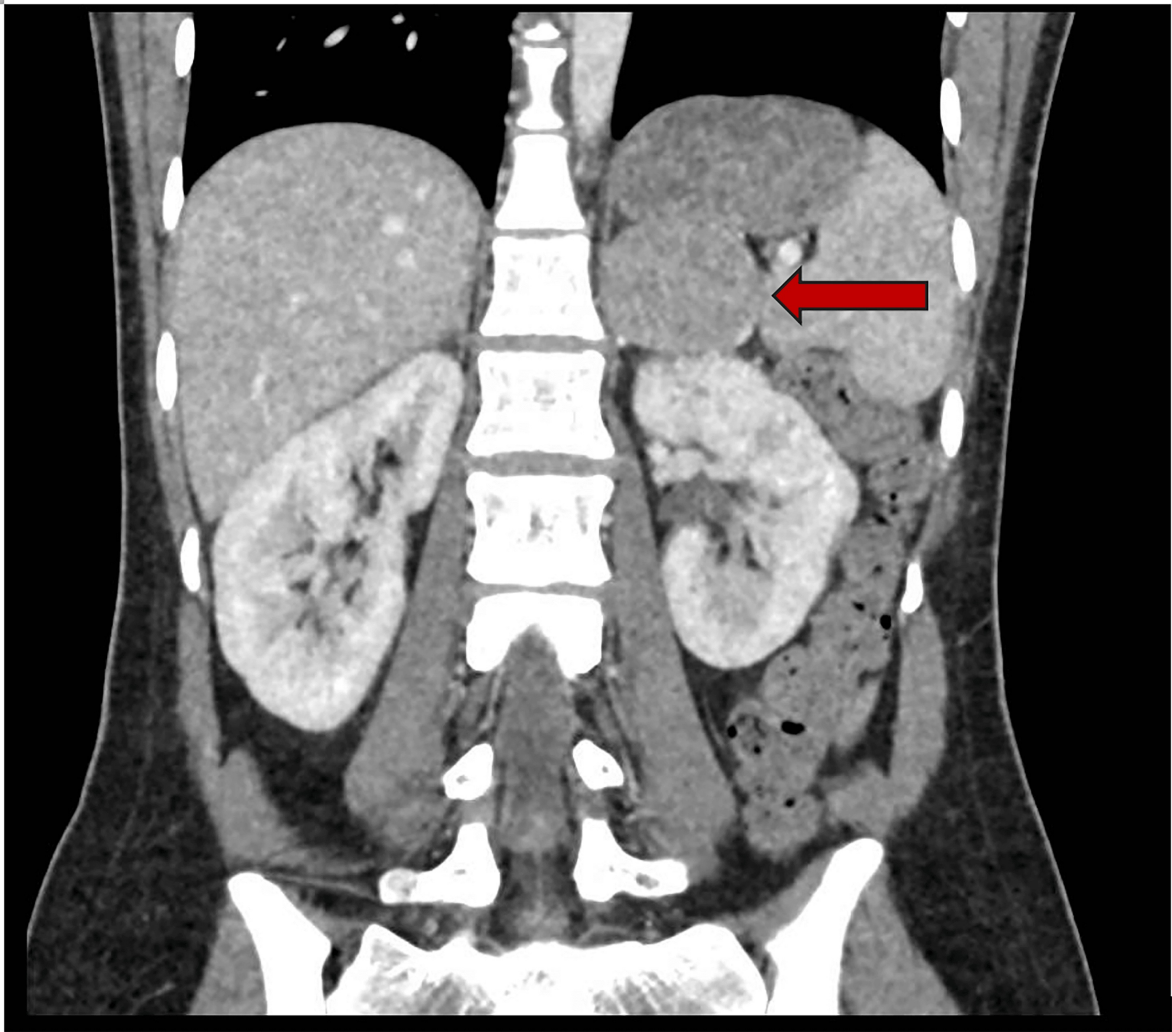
CT abdomen The red arrow points to the left adrenal mass (88 HU).
